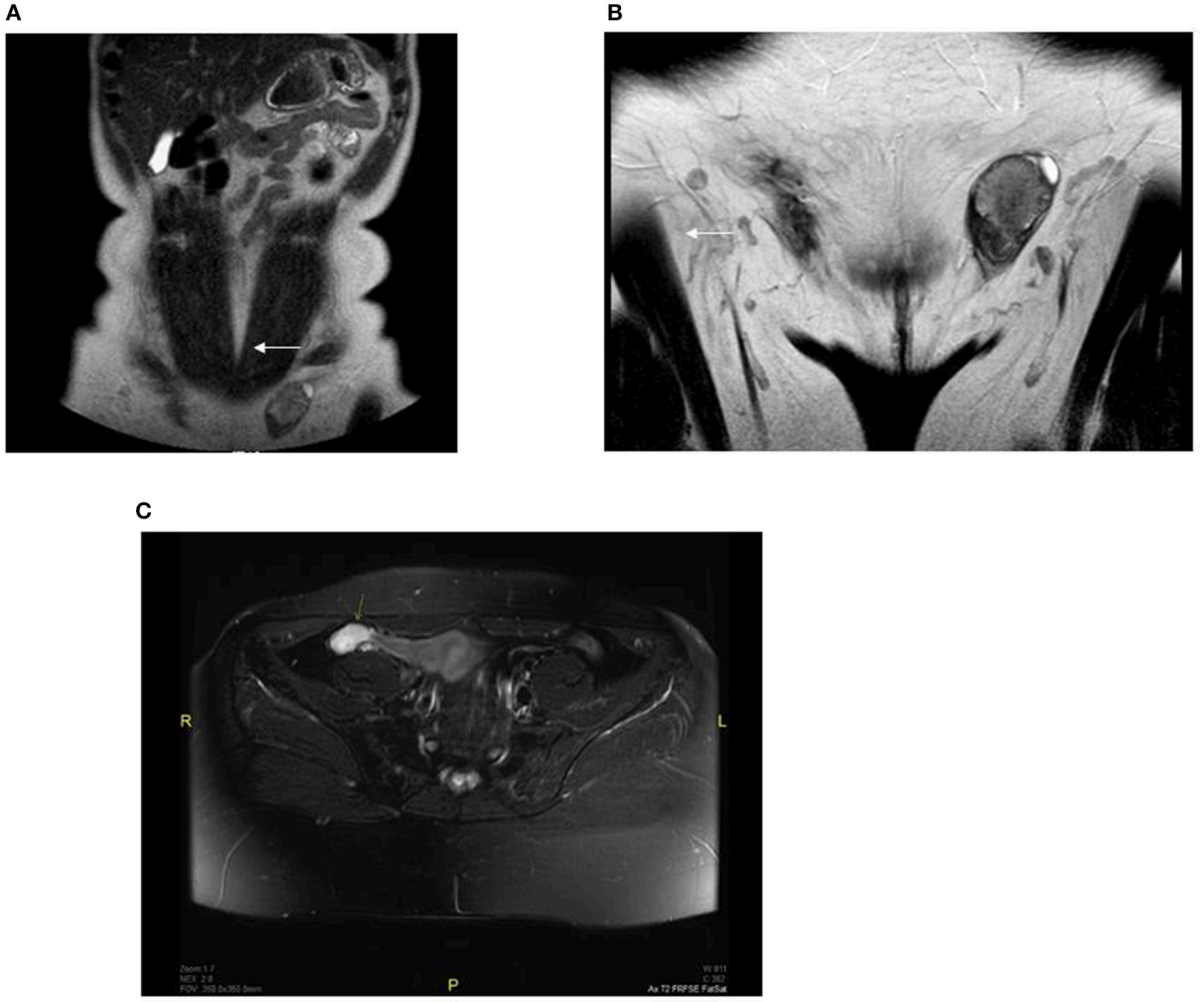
Preoperative MRI revealed oval structures without follicular activity: (A) right side (showed with an arrow) −1 × 1.3 cm, located near the anterior abdominal wall; (B) left side (showed with an arrow) −2.6 × 1.6 cm, located in the left inguinal canal; (C) pelvic MRI fat-suppressed T2 sequence of the right hypogastrium with a residual testicular structure 1.2 × 2.4 × 3.3 cm (showed with an arrow).
